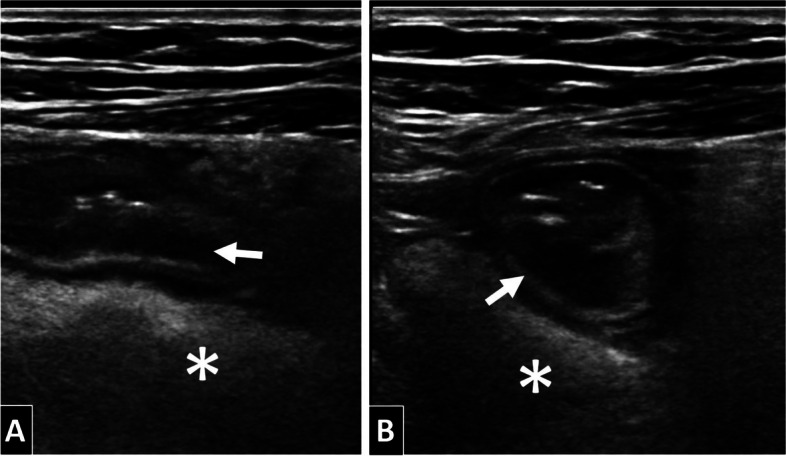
Patient of 62 years of age with ileal anisakiasis visible in ultrasound, sagittal (A) and axial view (B). The wall thickening can be observed due to oedema in the Kerckring valves (first hypoechogenic layer from the lumen, of submucosal location − white arrows), with important hyperechogenicity of the adjacent mesenteric fat (asterisks) due to severe oedema
